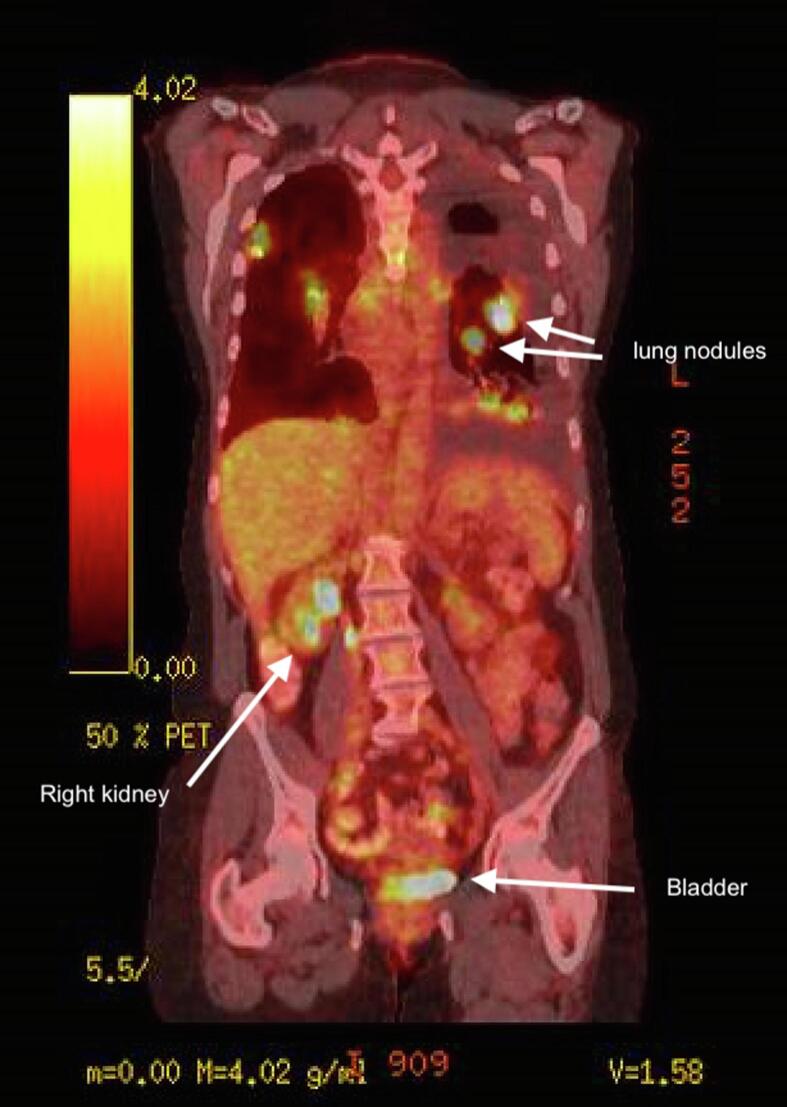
PET/CT scan demonstrating lung nodules and absence of abnormal abdominal and pelvic findings.
